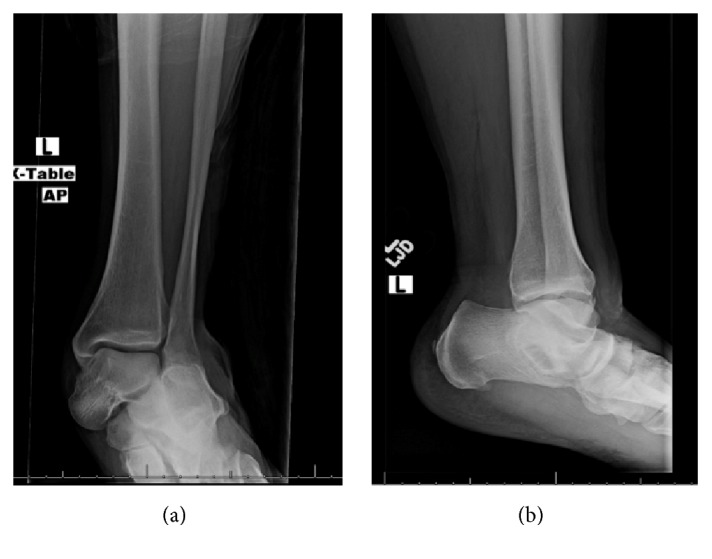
Injury radiographs demonstrating the subtalar dislocation.
